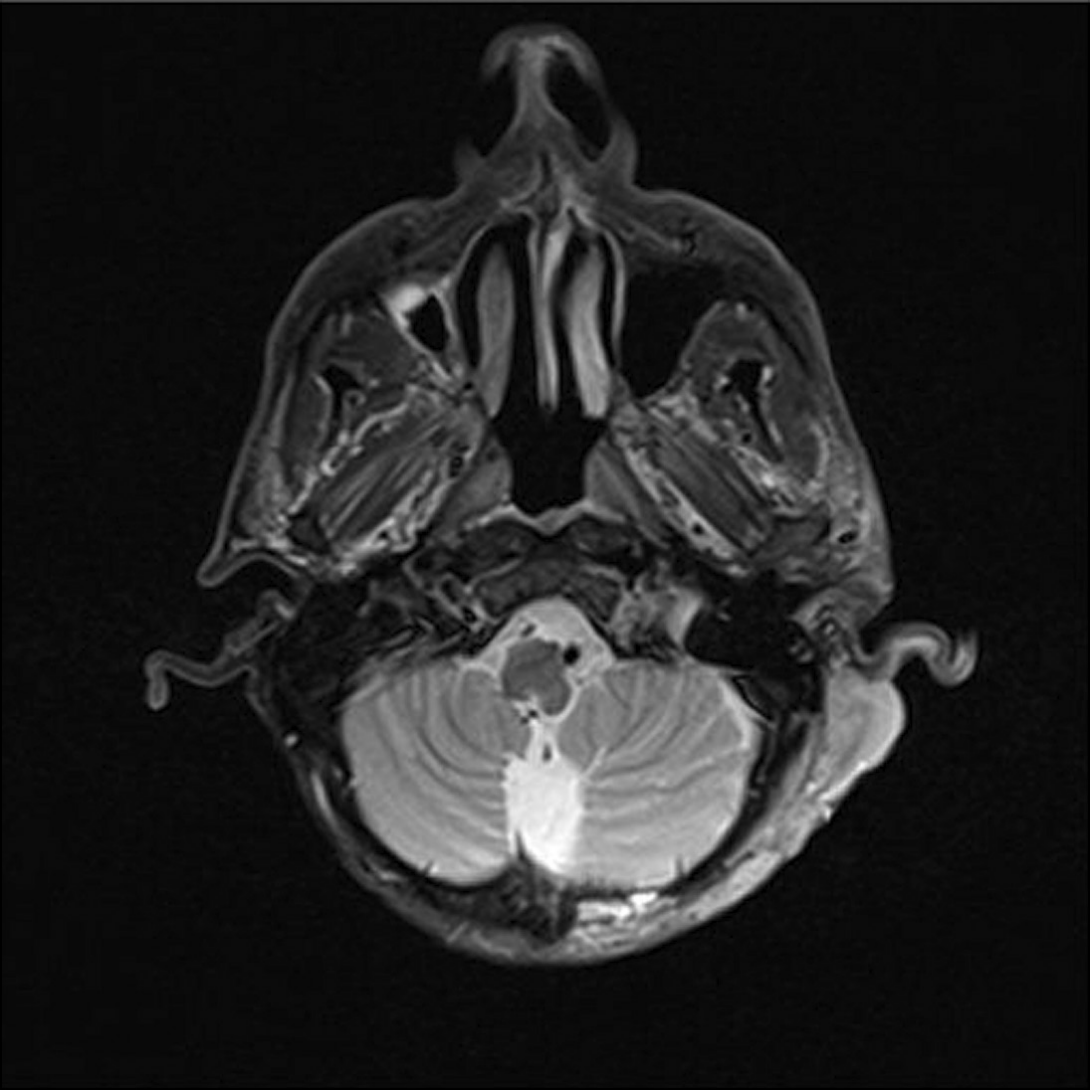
Axial T2W brain MRI shows uneven scalp thickening over the left posterolateral aspect of the posterior fossa, associated with bone destruction of the medial aspect of the left occiput with intracranial extra‐axial extension representing a malignant process including sarcoma
